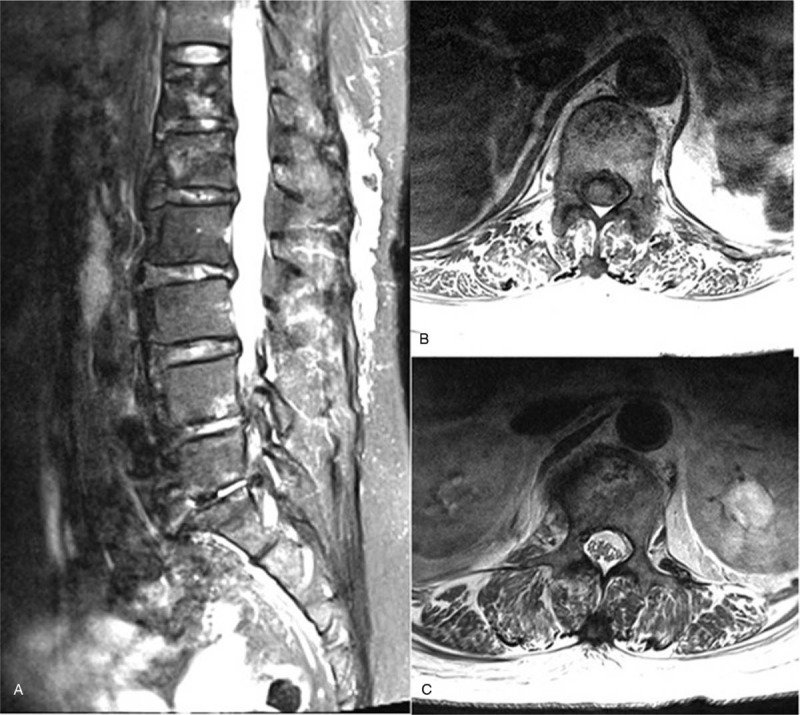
The enhanced T1 and T2 magnetic resonance imaging scan showing heterogenous signal intensity in the T12 (B) and L1 (C) vertebral bodies.
